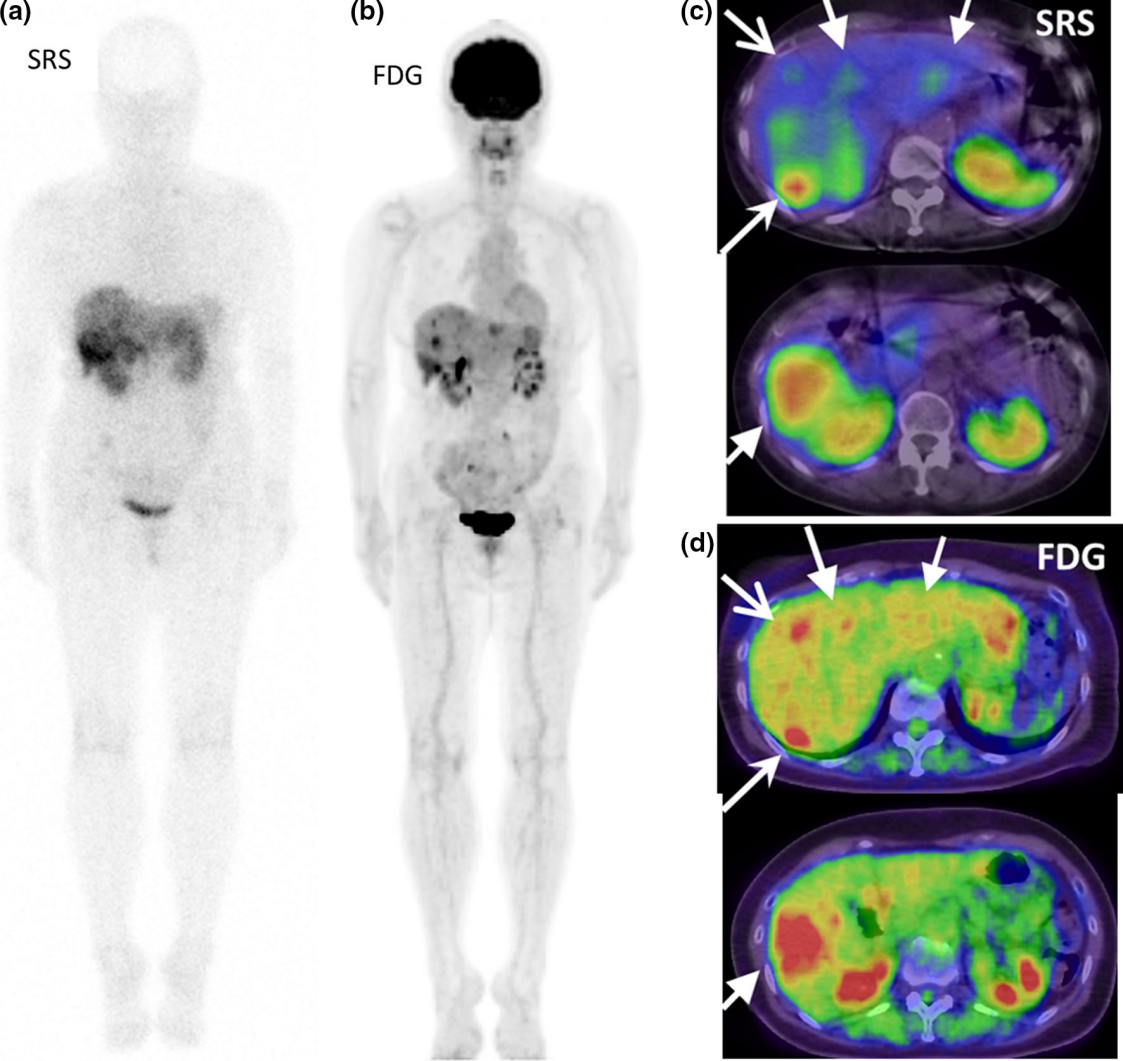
FDG-PET/CT images (b, d) and SRS images (a, c) of multiple liver metastases of G2 NET, unknown primary. Wholebody images (a, b) and two sets of axial images (c, d). Metastatic lesions in liver showed very large intra-patient variations in tracer uptake. Each arrow set in c and d indicated the same lesion seen in SRS and in FDG. Right posterior tumor showed high uptake both in FDG and in SRS, but right anterior tumor showed high uptake in FDG but low uptake in SRS
